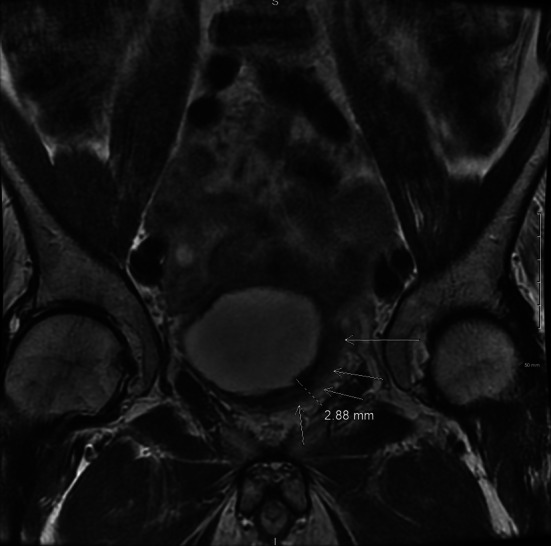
T2-weighted MRI images showed nonspecific irregular thickening of the left lateral bladder wall (arrows)
